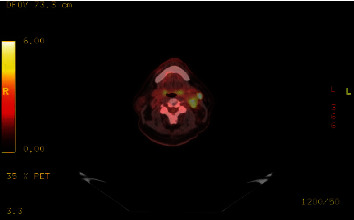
A hypermetabolic heterogeneous soft tissue mass at the left carotid bifurcation demonstrates SUV max of 7.1. This lesion is consistent with the given history of biopsy-proven paraganglioma. Multiple hypermetabolic left cervical lymph nodes are also seen (SUV max of 16.1).
